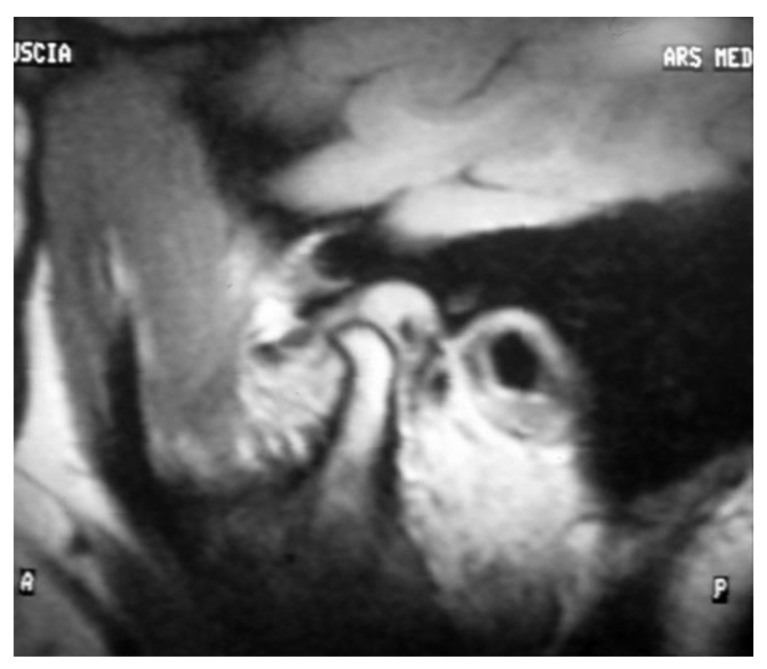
Magnetic Resonance Image before treatment T0. The MRI shows a joint effusion, degenerative bone alterations, disc displacement and remodeling.
